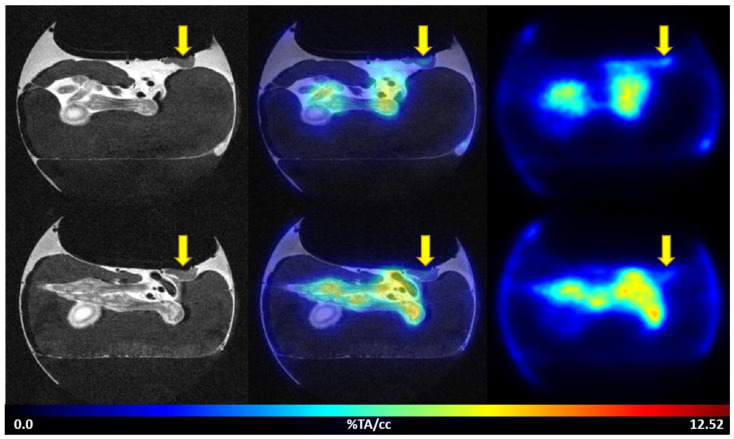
Representative images of [^68^Ga]Ga-Pentixafor PET/MRI in the HCT116 CAM-xenograft model. Upper row: baseline scan after i.v. injection of 7.19 MBq [^68^Ga]Ga-Pentixafor (0.79 nmol peptide) on EDD16. Lower row: blocking (315 µg AMD3100/egg) of [^68^Ga]Ga-Pentixafor uptake (18.32 MBq, 3.12 nmol peptide) in the same subject on the following day (EDD17). Left: T2-weighted TurboRARE images, right: [^68^Ga]Ga-Pentixafor PET 60 min p.i., middle: fused PET/MRI (axial planes are shown). HCT116 CAM-xenografts are marked with a yellow arrow.
